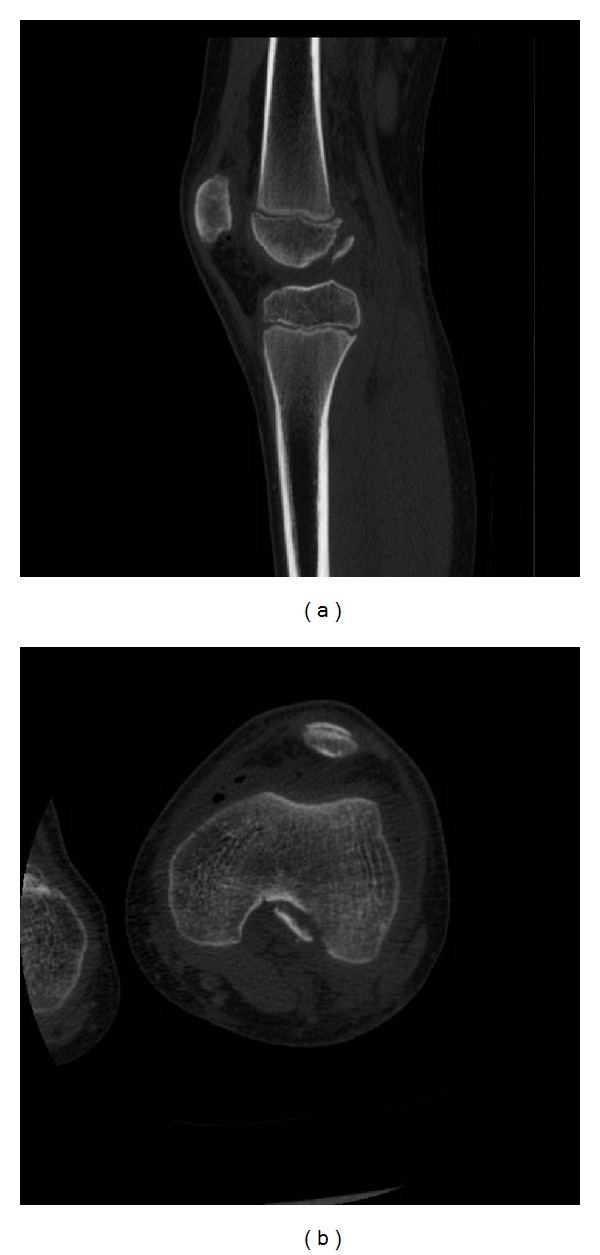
CT scan depicting (a) sagittal and (b) axial views of the patients knee depicting the avulsed osteochondral fragment.
